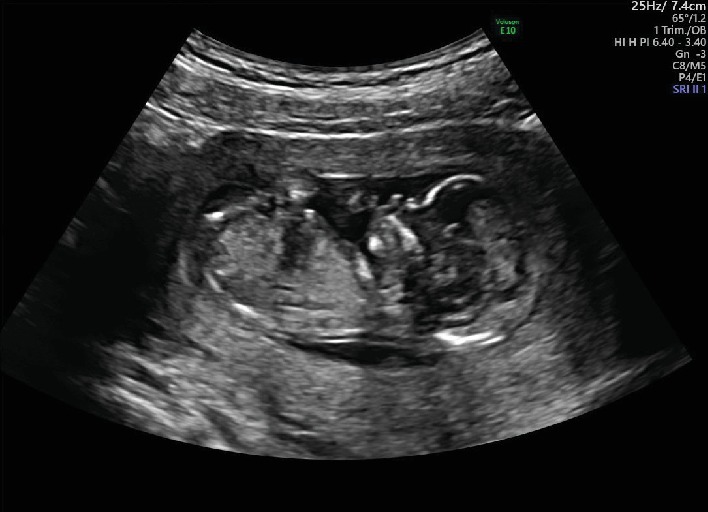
Gray-scale ultrasonography at the 13 weeks of gestation. Severe oligohydramnios was noted.
